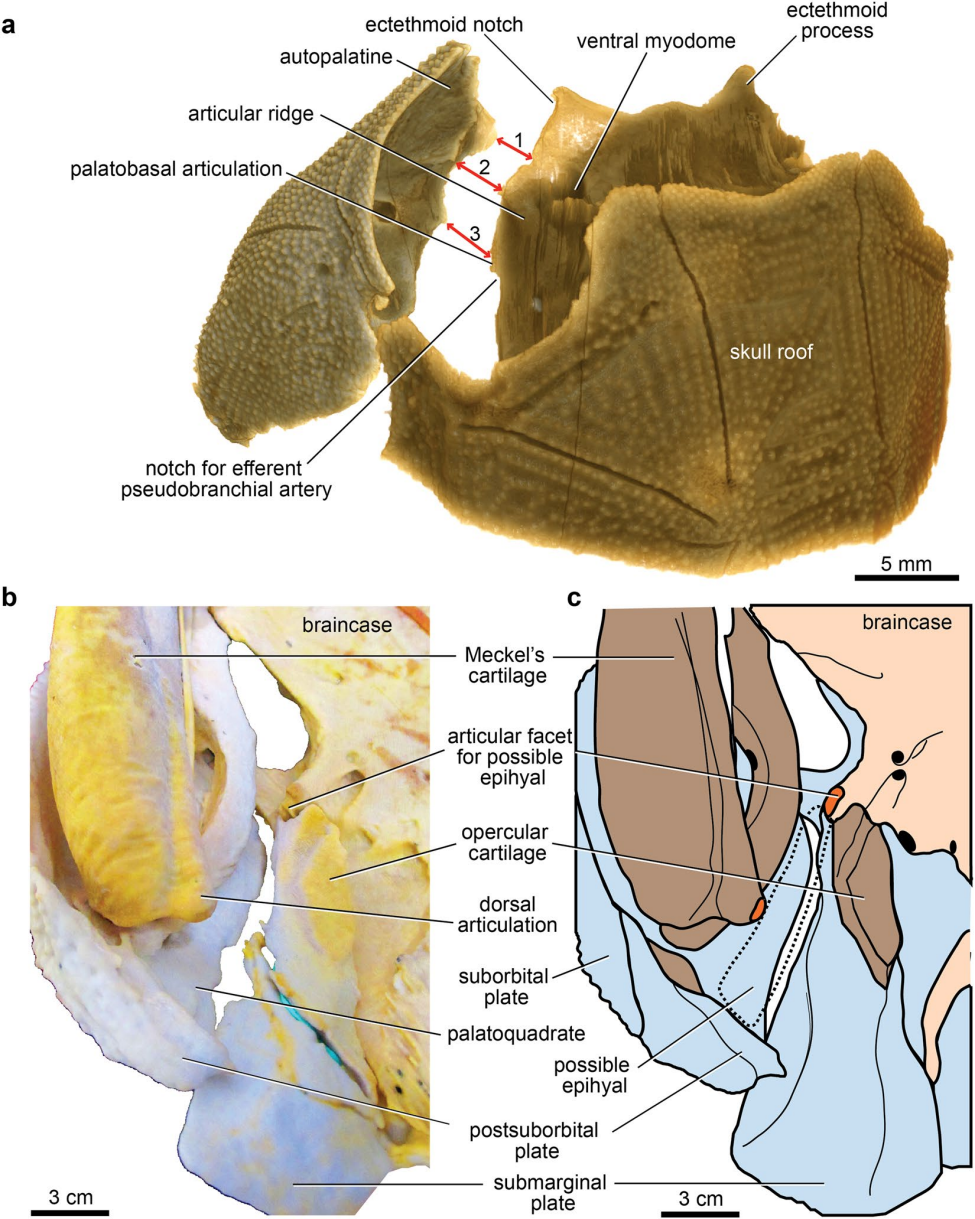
Jaw articulations of articulated buchanosteid arthrodire (ANU V244) based on high-resolution CT. (a) Dorsal view summarising articulations between the left autopalatine and subnasal shelf of the braincase (red arrows: 1, mesial articulation of ethmoid connection; 2, lateral articulation of ethmoid connection; 3, palatobasal connection). (b) Ventral view of assembled 3D printouts of right Meckel’s cartilage, palatoquadrate, suborbital and postsuborbital plates in position against the skull and braincase. (c) Reconstruction based on (b). Light blue, dermal bones; brown, visceral arch cartilages; pale pink, braincase; orange, articular facets. 3D printouts are 6 times natural size.
